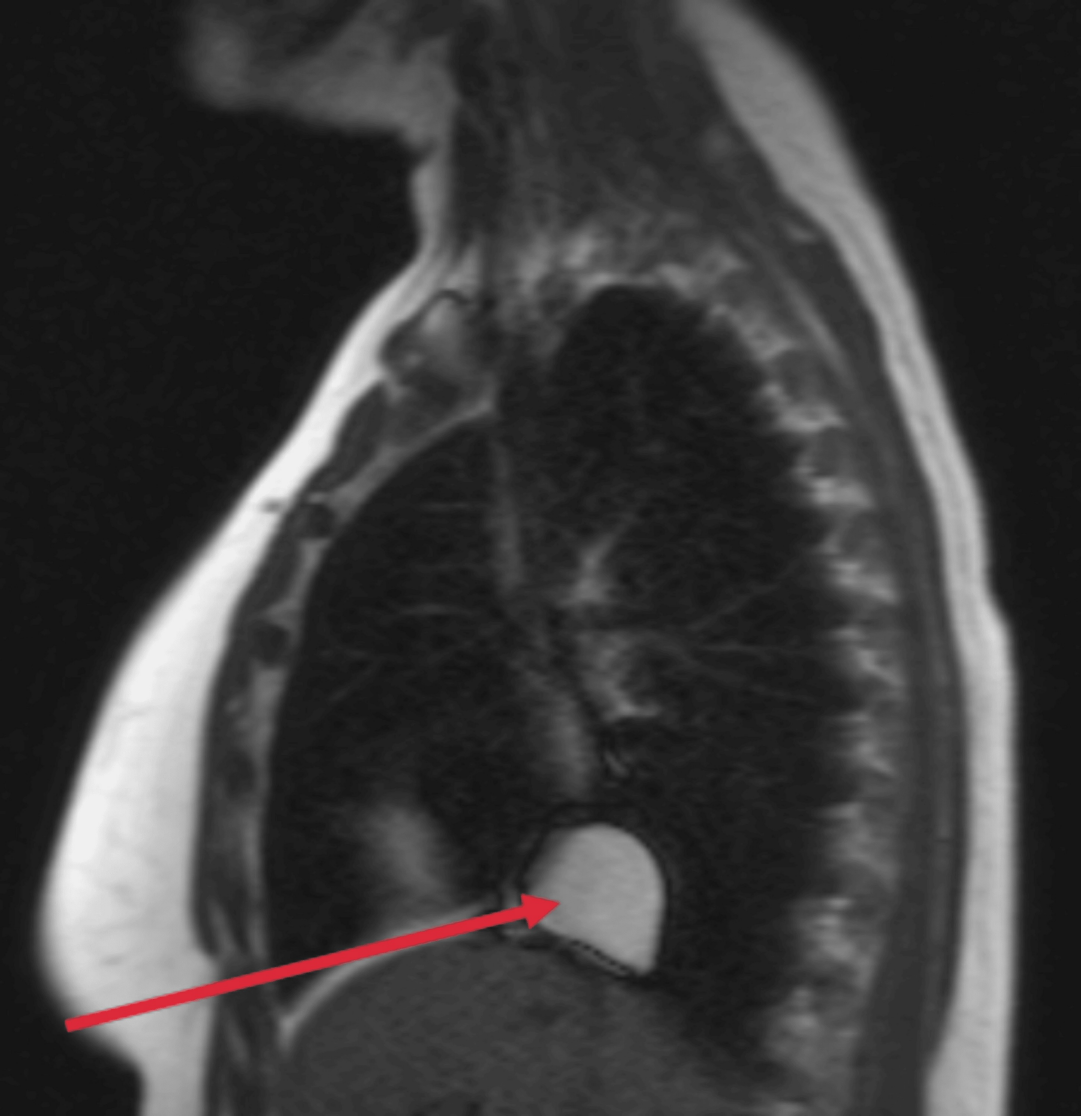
Sagital view of the cardiac MRI. The red arrow points to the pericardial cyst.
